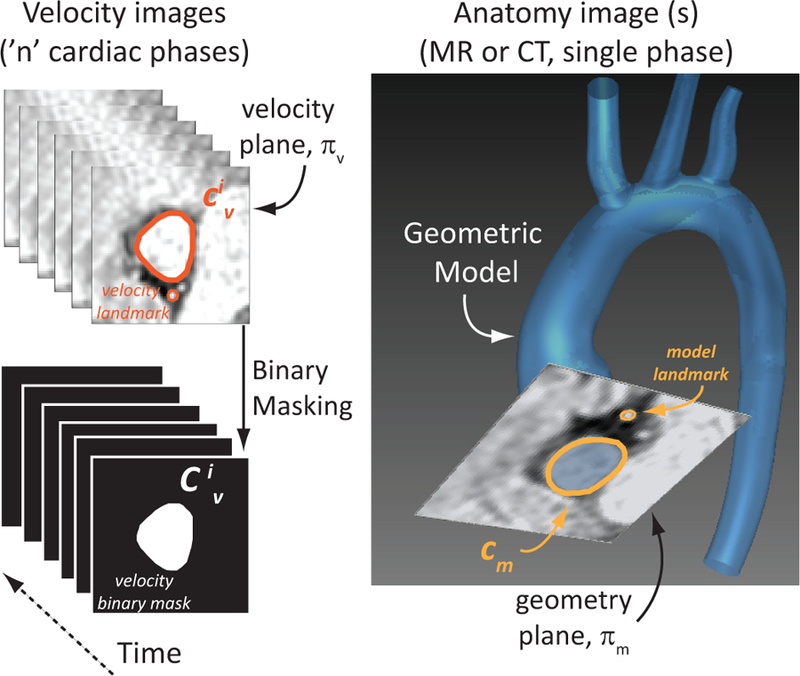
Method overview. Through-plane velocity is extracted from 3D colour Doppler or 2D flow MRI data (left). For each temporal phase *i* in the velocity data, a velocity contour $c_{\upsilon }^{i}\subset \pi_{\upsilon }$ defining the boundary of the vessel is obtained (left). A separate model contour $c_{m}\subset \pi_{m}$ is defined in the face of the geometric model, built from the anatomy image data (CT or MRI; right). In this work, $c_{m}$ is not time-dependent, but this need not be the case in general. In order to co-register $c_{\upsilon }^{i}$ and $c_{m}$, the user must define landmarks in both velocity and anatomy images. The velocity profiles between each of the *n* cardiac phases are temporally interpolated to produce the required resolution for the CFD analysis
